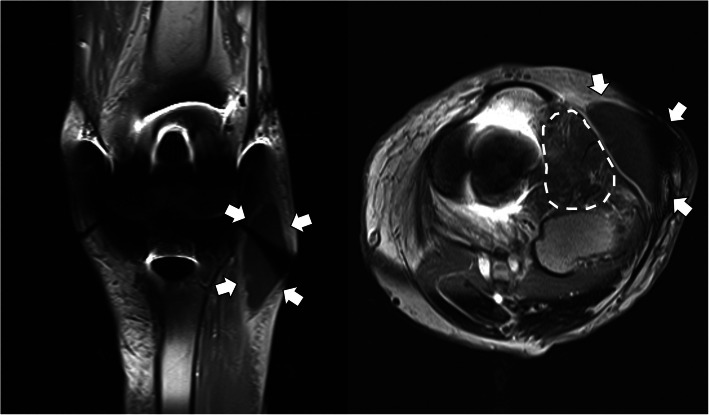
Contrast-enhanced T1-weighted magnetic resonance images of the left knee, showing destruction of the lateral tibial condyle filled with granulation tissue (dotted line) extending to adjacent subcutaneous abscess lesion (arrows) on the anterolateral aspect of the knee joint
